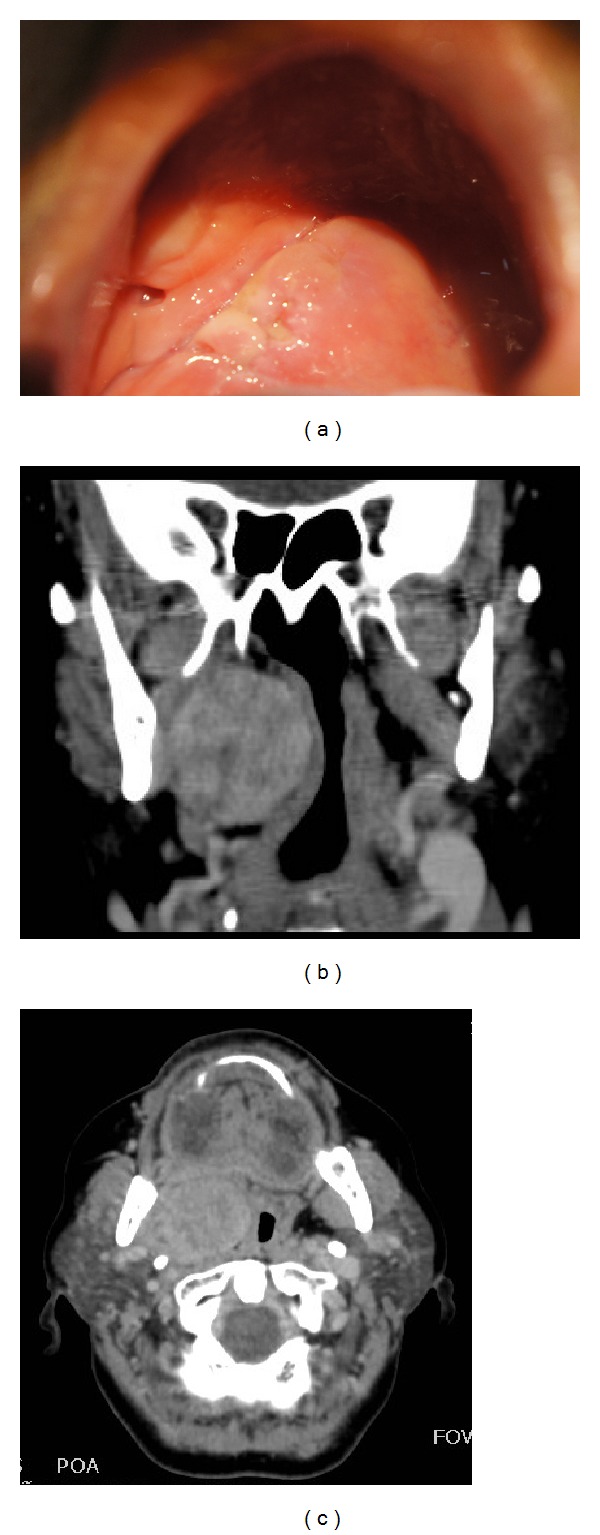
Nodular lesion on right side of soft palate lined by normal mucosa (a). Axial (b) and coronal (c) computed tomography scans showing a hyperdense expansive lesion with well-defined limits measuring 3.7 × 3.3 cm, determining compression and displacement to the left part of the nasopharynx and oropharynx, and causing narrowing of the airways.
